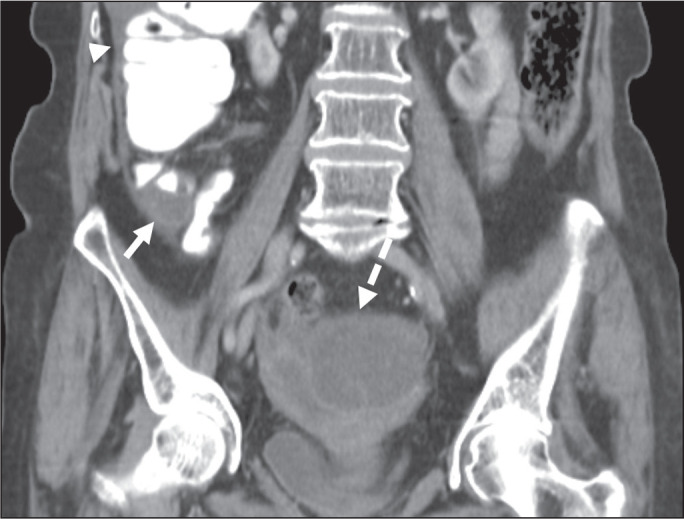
LAMN with PMP and ovarian involvement. Contrast-enhanced coronal CT
